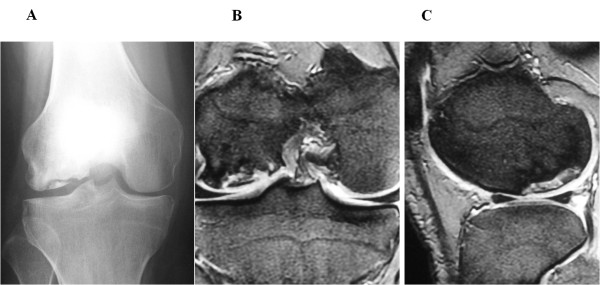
Preoperative findings in case 1. (A) Anteroposterior radiograph reveals an osteonecrotic lesion in the right lateral femoral condyle. (B,C) Coronal and saggital MRI shows an osteonecrotic lesion with high signal change on the T2-weighted image in the right lateral femoral condyle.
